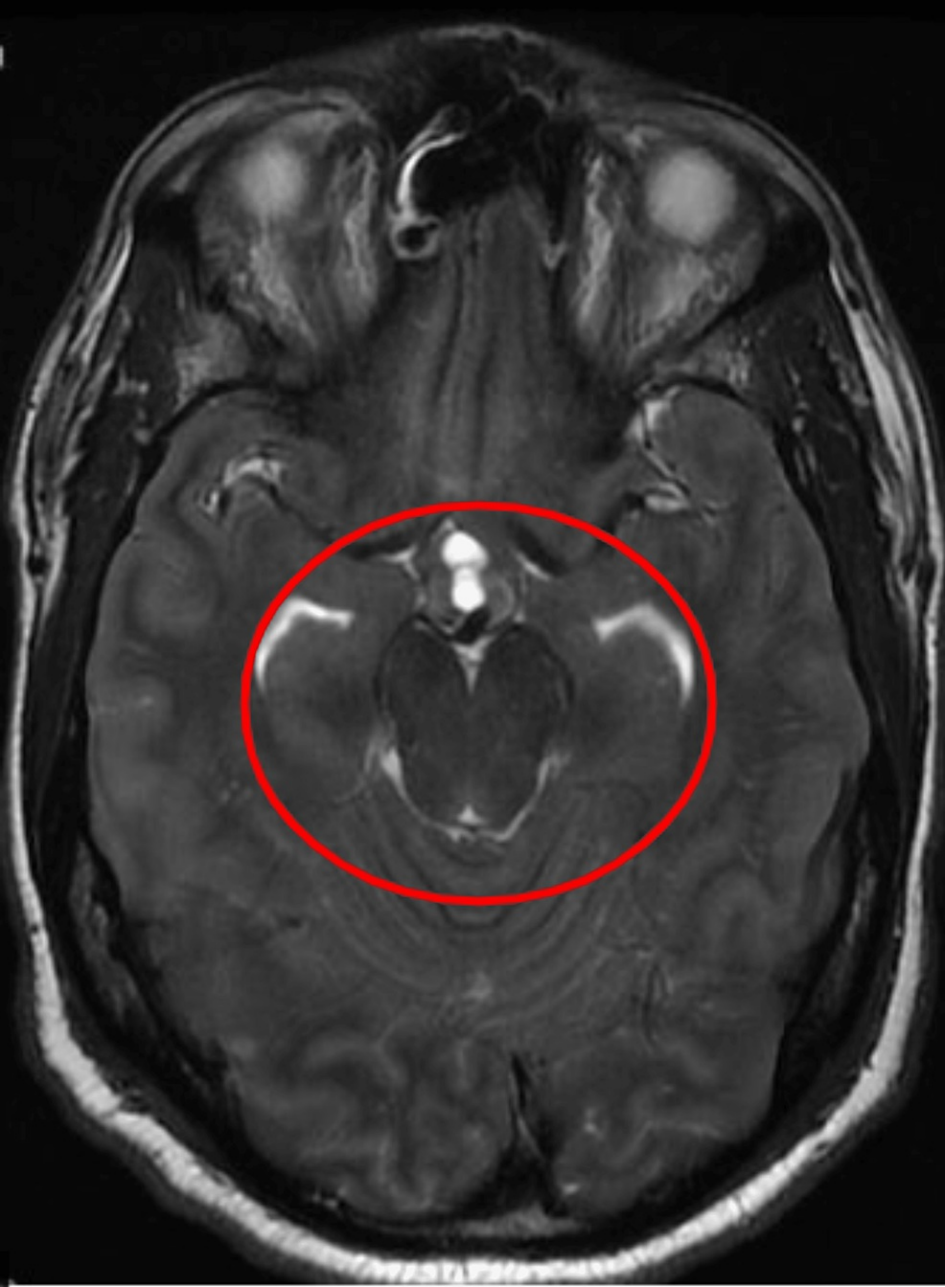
MRI brain T2 weighted series showing crowding of the cisterns suggesting herniation
